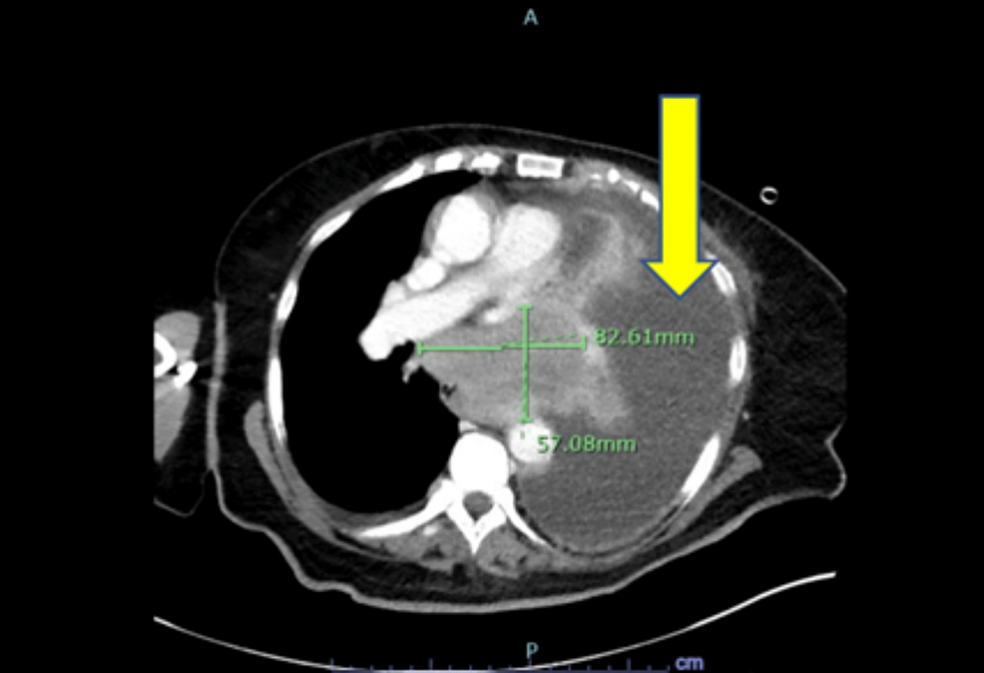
Chest CT showing huge mediastinal mass (measurements as noted) along with massive left-sided pleural effusion (arrow)
